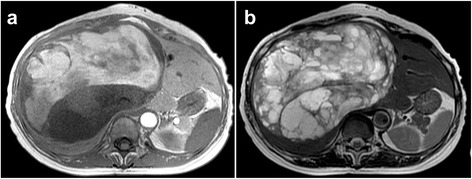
MRI revealed high and low signal intensity in T1-weighted images (a) and high signal intensity in T2-weighted images (b)
